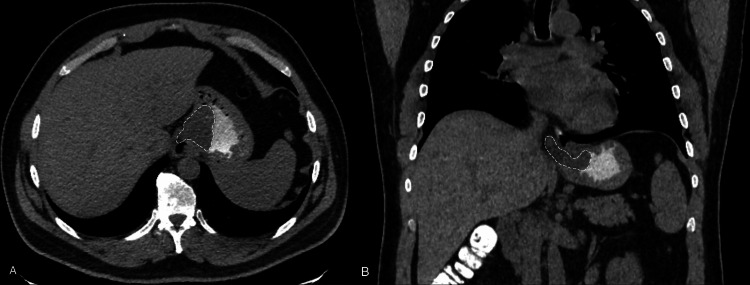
Axial (A) and coronal (B) abdominal CT scan: the highlighted area demonstrating a well-defined mass in the distal esophagus protruding into the lesser curvature of the stomach.
